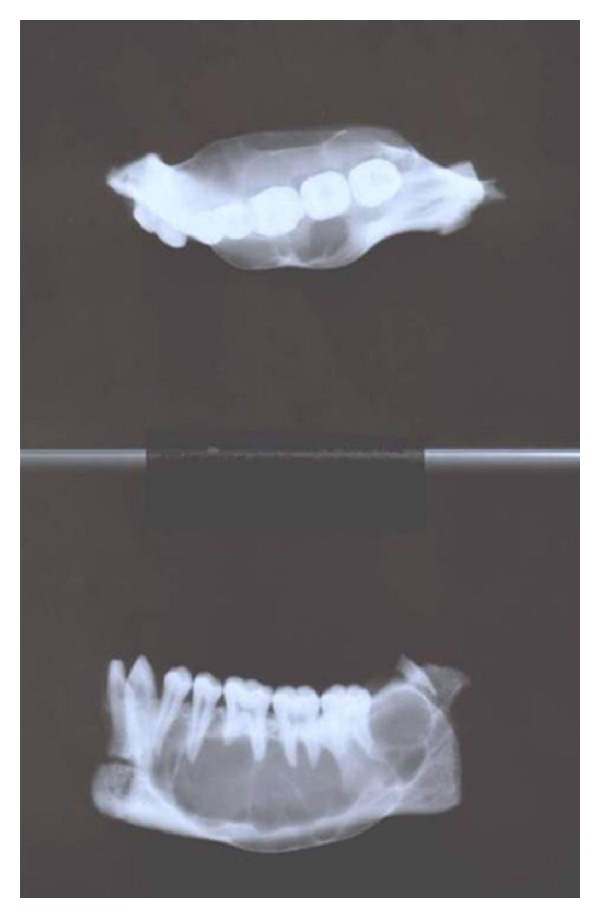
Radiograph of resected segment of right-side mandible.
